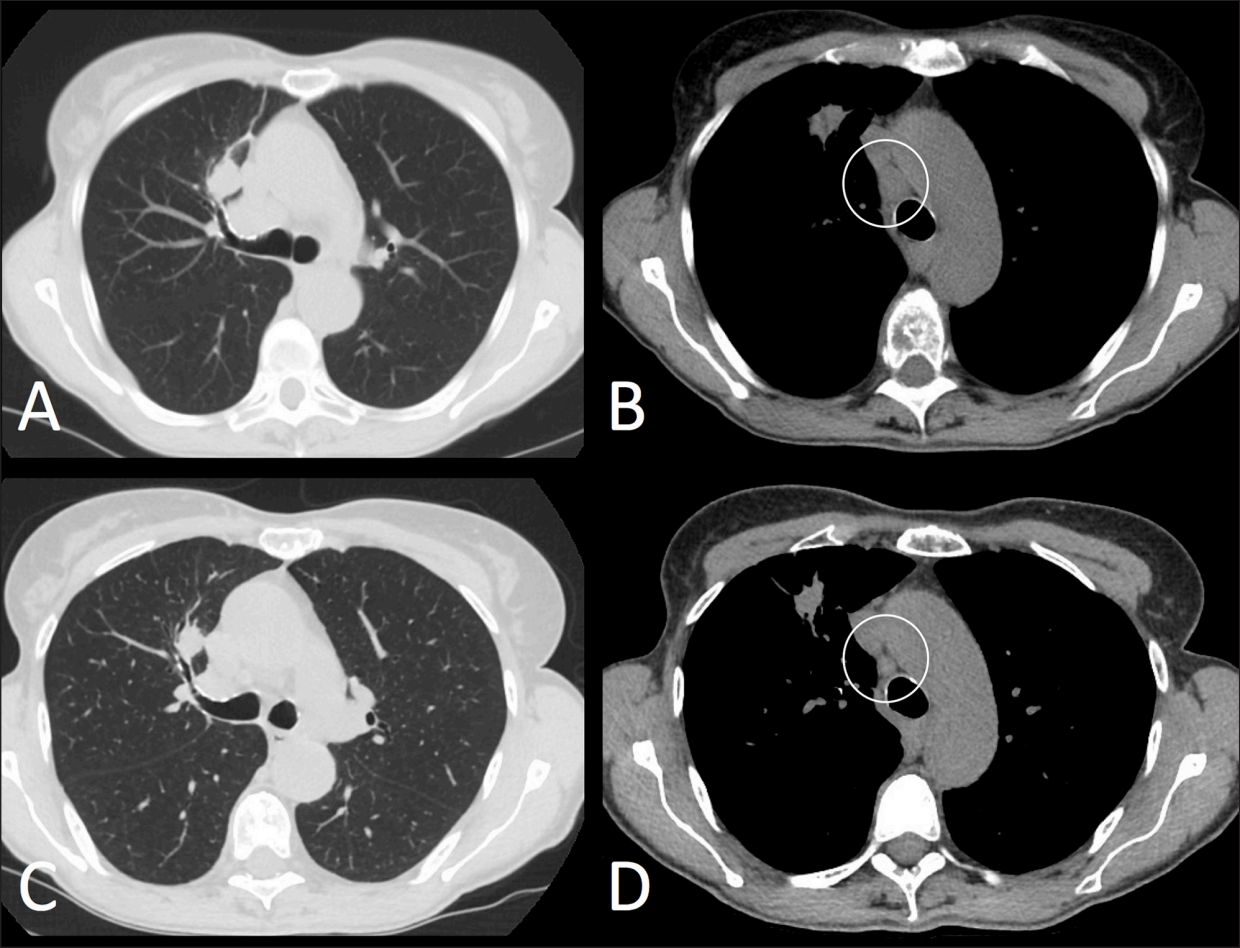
AB = baseline, CD = eight weeks follow-up. Axial non-contrast enhanced CT-images in lung and mediastinal window settings show a decrease in size of the primary lung tumor in the right upper lobe (A, C). Also note the decreased short axis of the mediastinal adenopathies (B, D). Regarding the extra-osseous lesions, patient would have been classified according to RECIST 1.1. as partial response.
